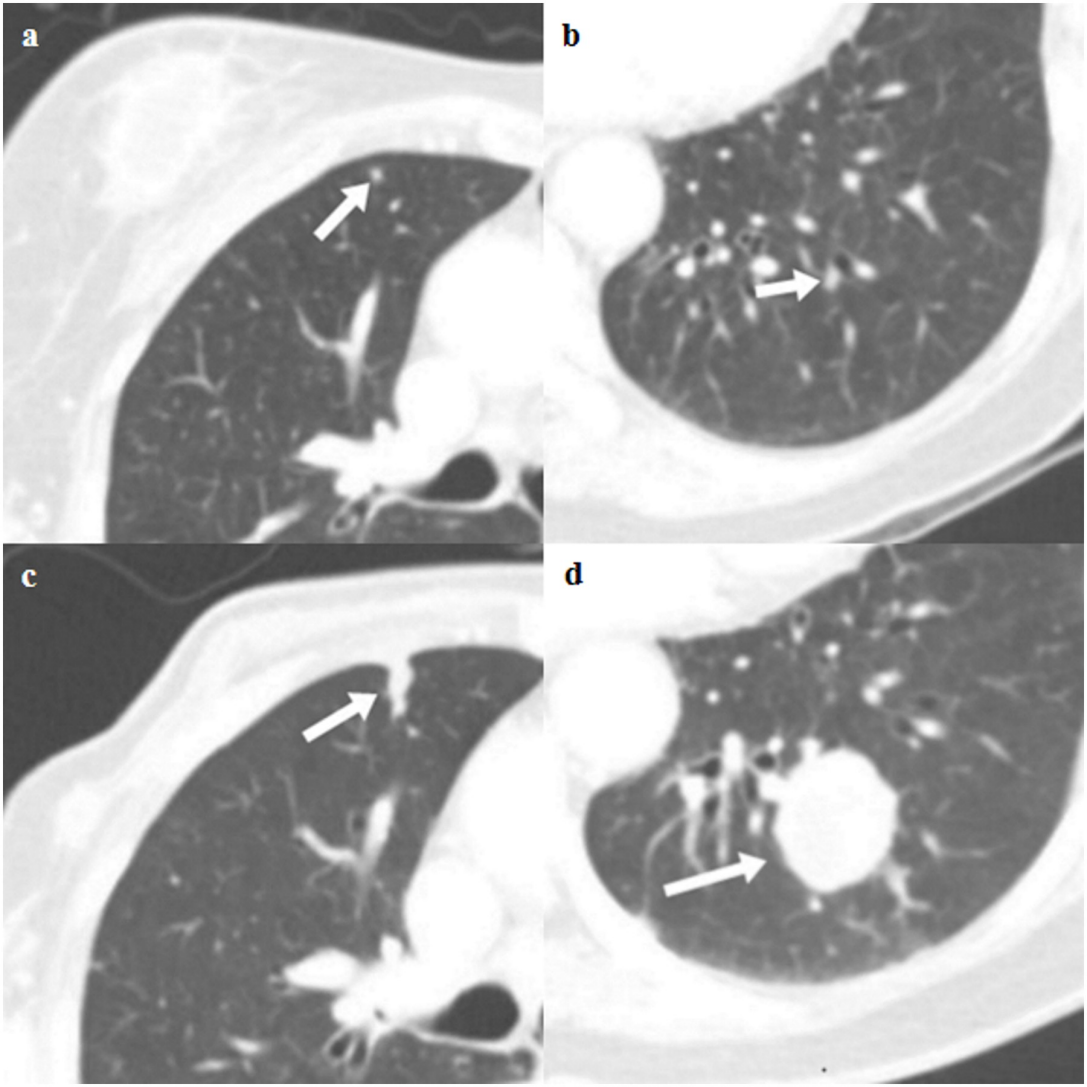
Axial CT images of true lung metastases in a 78-year-old female with pathologic stage III breast cancer. (a and b) Staging chest CT images show two small nodules (arrow) in the right middle lobe and left lower lobe (indeterminate). (c and d) Follow-up chest CT images show growth of two nodules (arrow), which are thought to be pulmonary metastasis.
